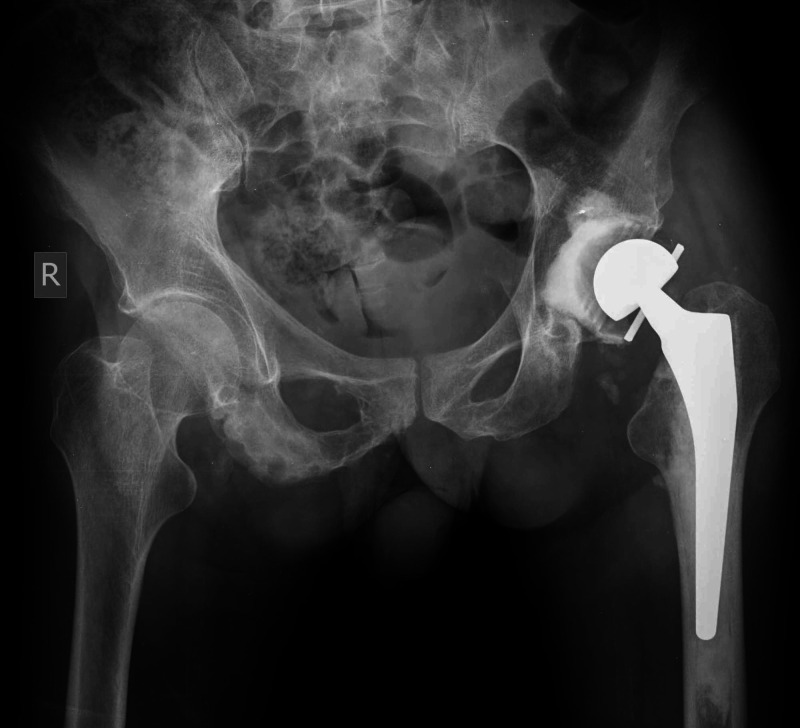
Postoperative anteroposterior pelvis radiograph showing revision of hemiarthroplasty of the left hip to a total hip arthroplasty with cemented constrained liner
